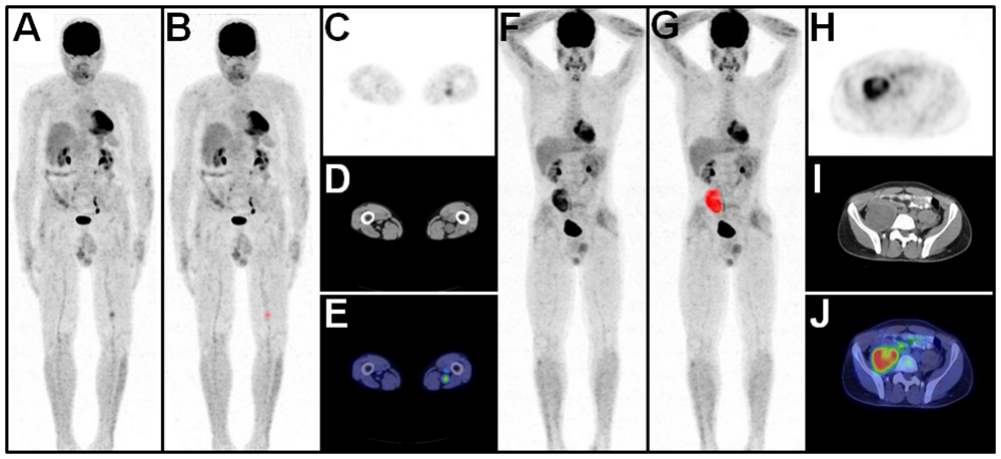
Solitary BPNST versus solitary MPNST. Maximum intensity projection PET image (A) of a patient with a solitary BPNST (SUV_max_ / MTV / TLG: 2.6 / 2885 / 6488) in the left thigh with automatically delineated metabolically active tumors (*red*) (B), corresponding axial PET (C), CT (D) and fused PET/CT image (E). Maximum intensity projection PET image of a patient with a solitary MPNST (SUV_max_ / MTV / TLG: 7.1 / 171328 / 633913) in the right pelvis (F) with automatically delineated metabolically active tumors (*red*) (G) with corresponding PET (H), CT (I) and PET/CT image (J).
